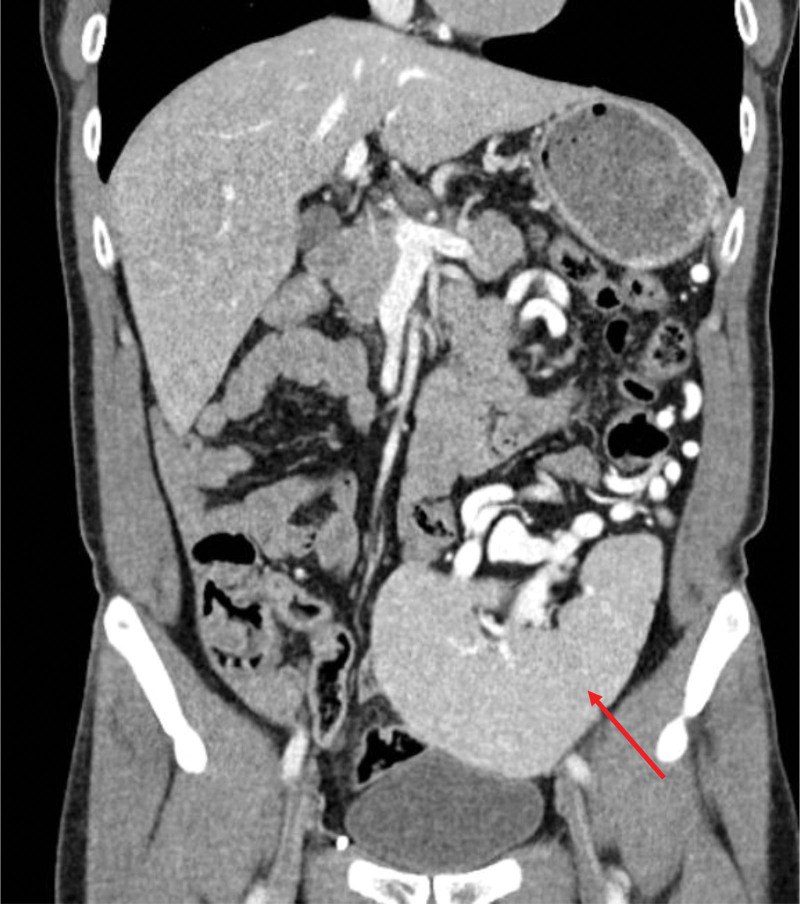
Initial abdominal CT performed on December 12, 2023 (hospital day 1) showing a large volume of hypodense material filling the stomach lumen, suggesting recent upper gastrointestinal bleeding. The spleen is abnormally located in the left pelvic cavity (arrow), consistent with a wandering spleen. Engorged perigastric collateral veins are visualized in the absence of cirrhotic changes. CT = computed tomography.
